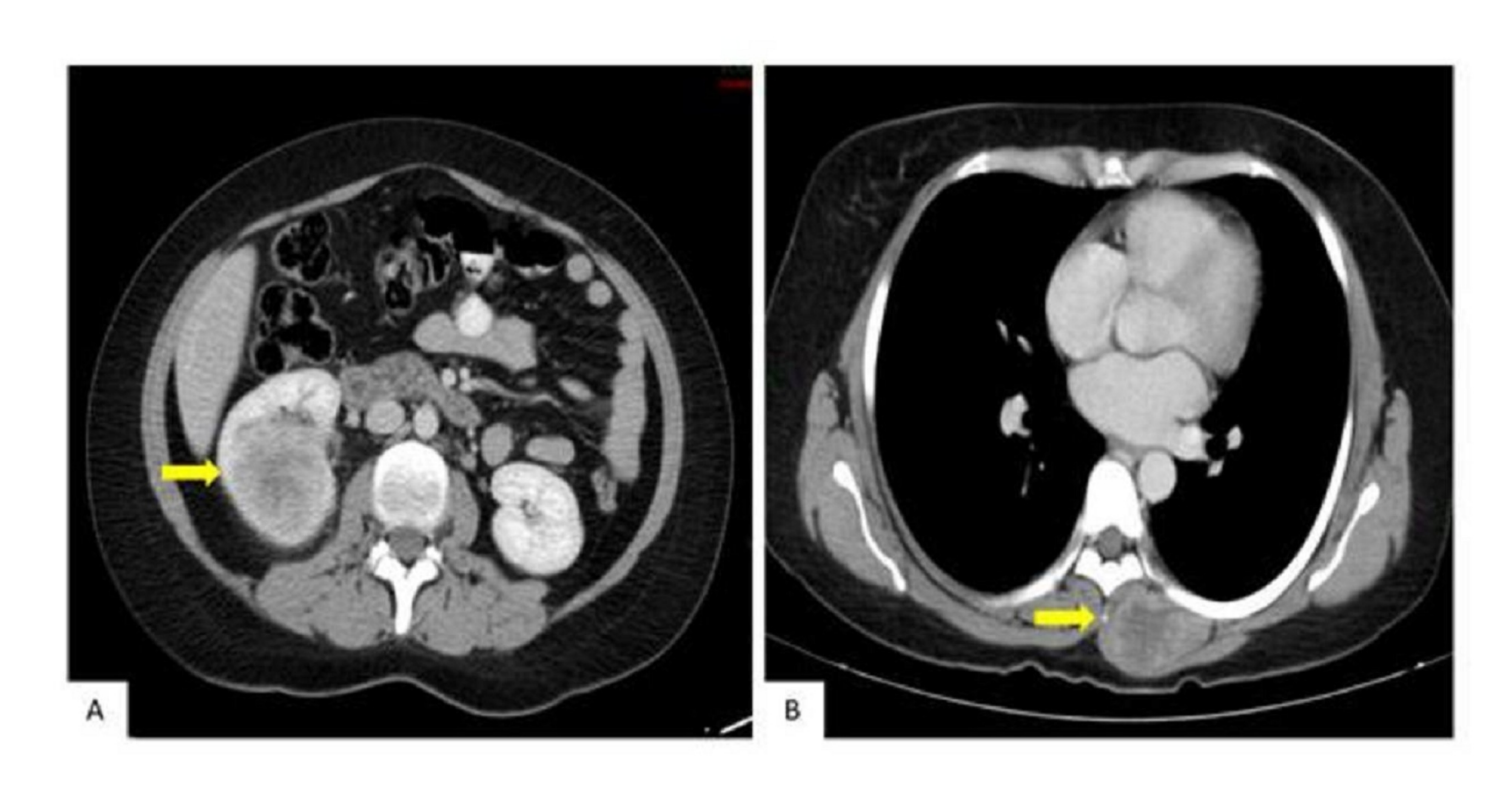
Abdominal and Thoracic CT Scan A: Axial computed tomography (CT) scan of the abdomen showing a predominantly heterogeneous right renal mass with areas of necrosis. B: Thoracic CT scan in axial section showing a mass in the left paraspinal musculature, with signs of necrosis.
